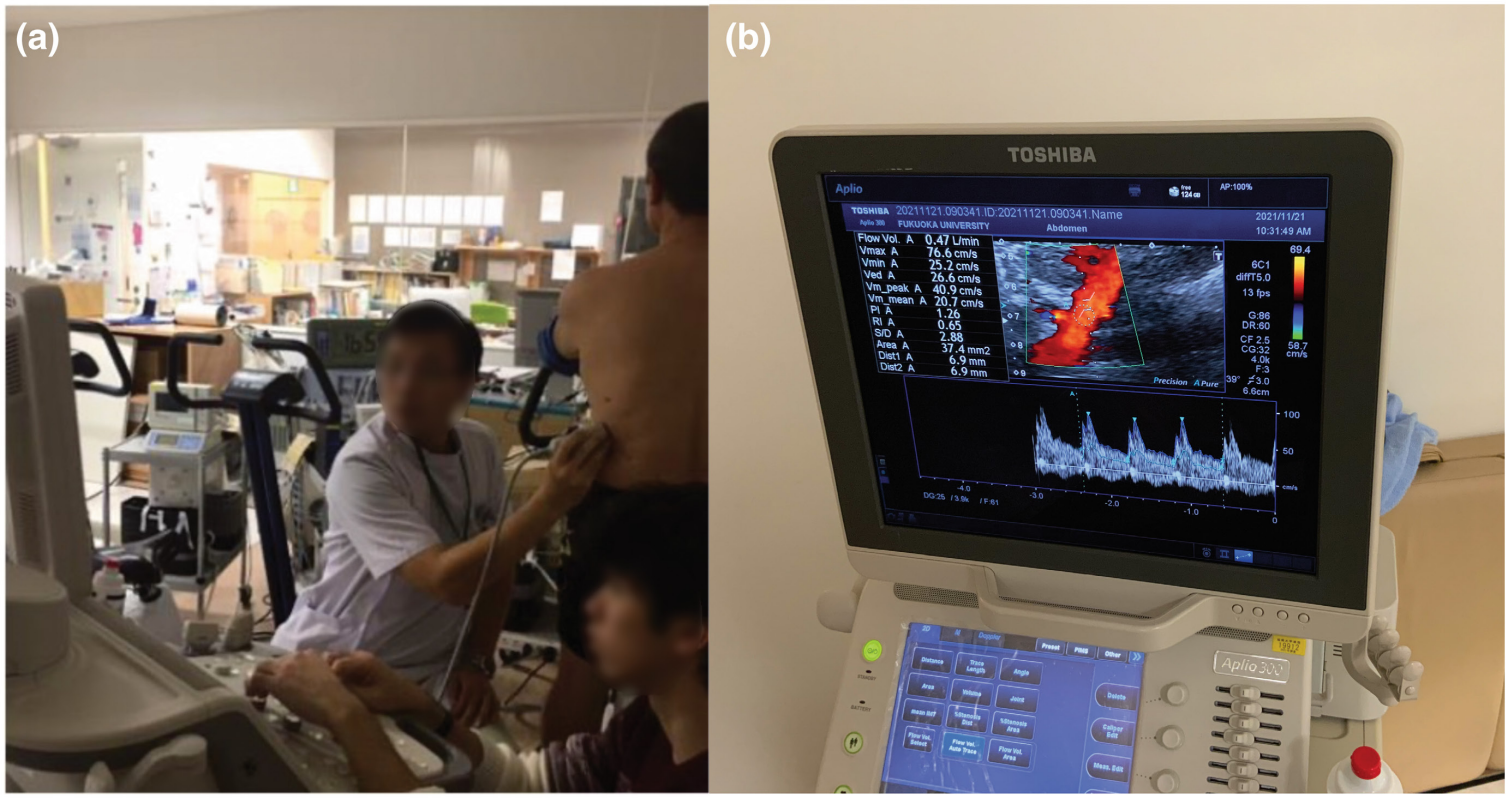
Experimental set‐up and Doppler ultrasound imaging of the kidney. (a) measuring scene, the technician placed the probe on the left lumbar region of the participant in a seated position. (b) The renal artery is drawn, and the technician moved the cursor to the renal artery and measured blood flow velocity (BFV) using the pulse Doppler method, with the average of three Doppler waveforms defined as the mean blood flow waveform. Cross‐sectional area (CSA) is then determined, and renal blood flow (RBF) is calculated based on BFV and CSA using the following equation: RBF (mL/min) = BFV (cm/s) × CSA (mm^2^) × 60 (s). The value for Flow Vol (i.e., renal blood flow), Vmax (i.e., peak systolic flow velocity), Ved (i.e., end‐diastolic flow velocity), Vm_mean (i.e., blood flow velocity), renal pulsatility index (rPI), renal resistive index (rRI), Area (i.e., cross‐sectional area), and Dist (i.e., diameter) are presented.
